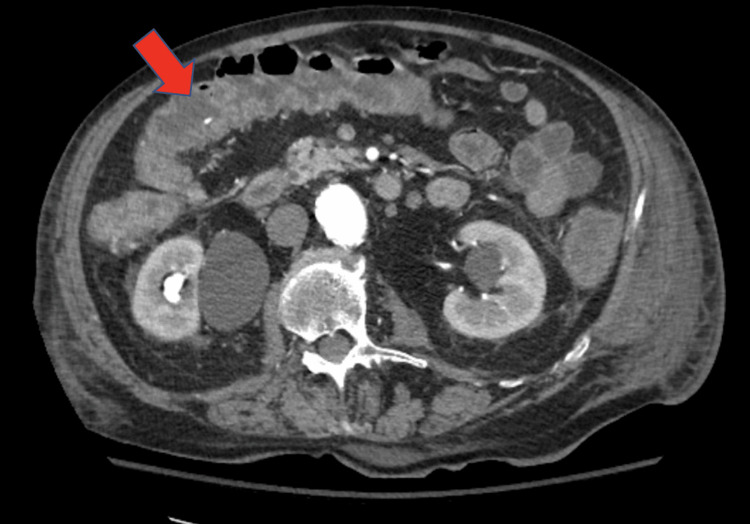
A repeat CT abdomen and pelvis on hospital day 9, which revealed worsening inflammatory changes in the sigmoid, descending, and ascending colon suggestive of colitis.
